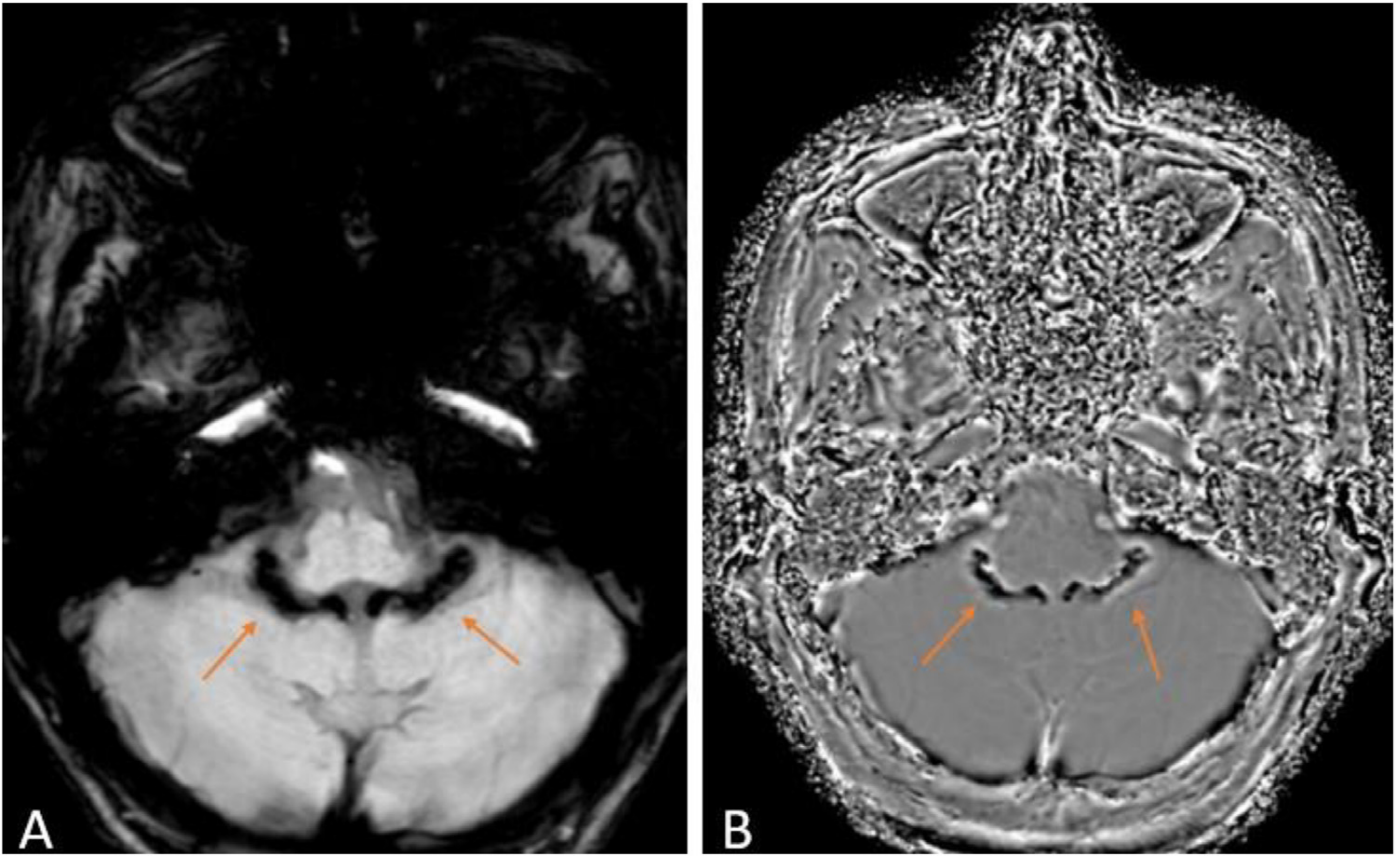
Magnetic resonance imaging of the brain of a 28-year-old chronic alcoholic with a history of loss of consciousness after sleeping in the night, axial section susceptibility-weighted imaging phase (A) and susceptibility-weighted imaging magnitude (B), showing Bochdalek's flower basket – a normal anatomical variant of choroid plexus (orange arrows).
